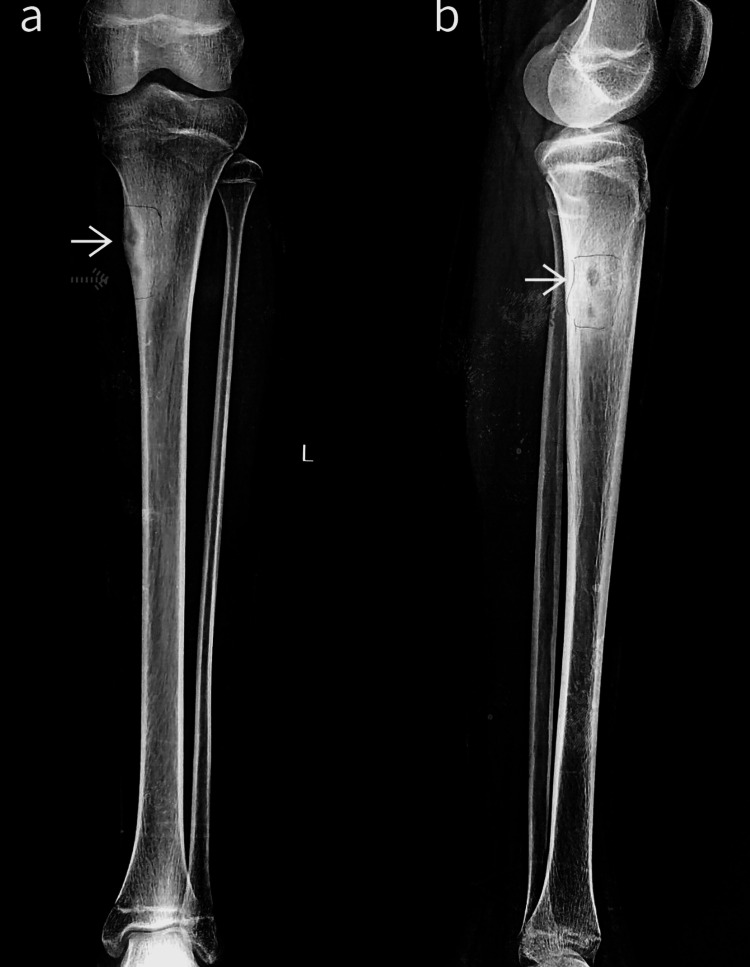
Anteroposterior (a) and lateral (b) plain radiographs of the left knee with the leg ankle Two lytic bone lesions with surrounding reactive sclerosis within the proximal meta-diaphyseal region of the medial tibial cortex (white and dotted arrows showing two nidi).
